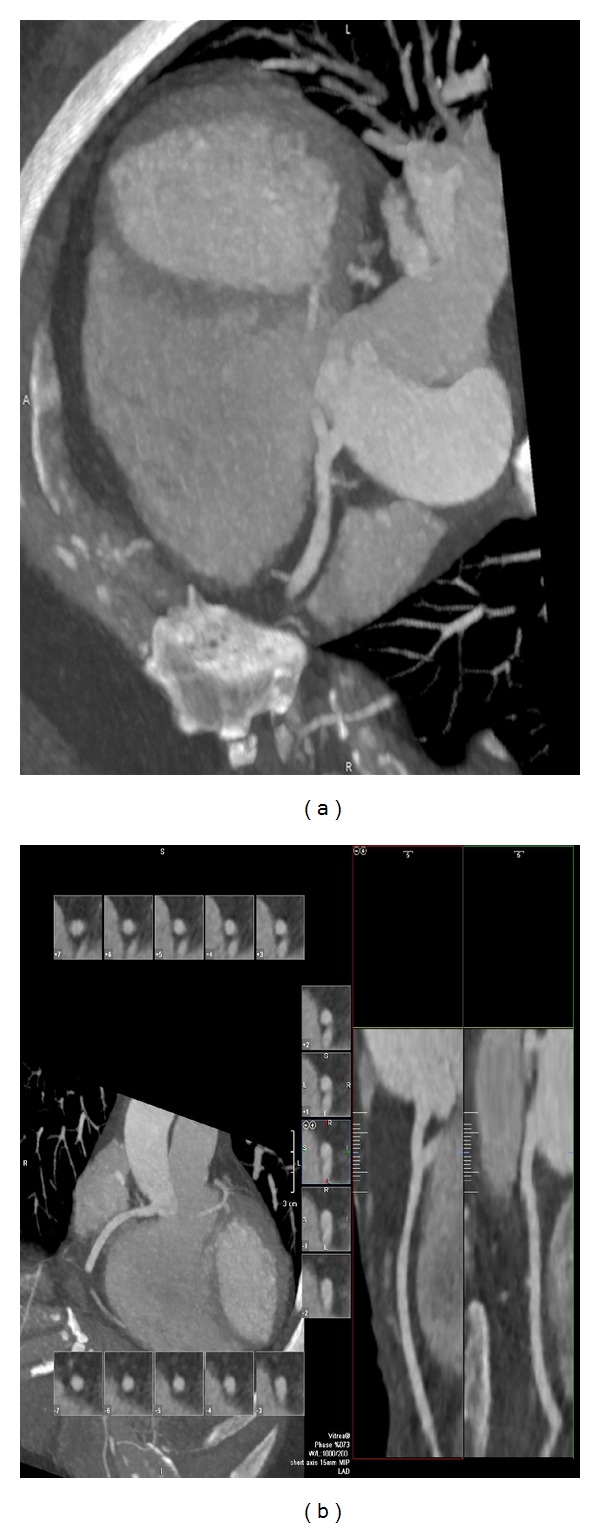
Cardiac computed tomography showing anomalous left main off of the right sinus of Valsalva and subsequent intraseptal course.
